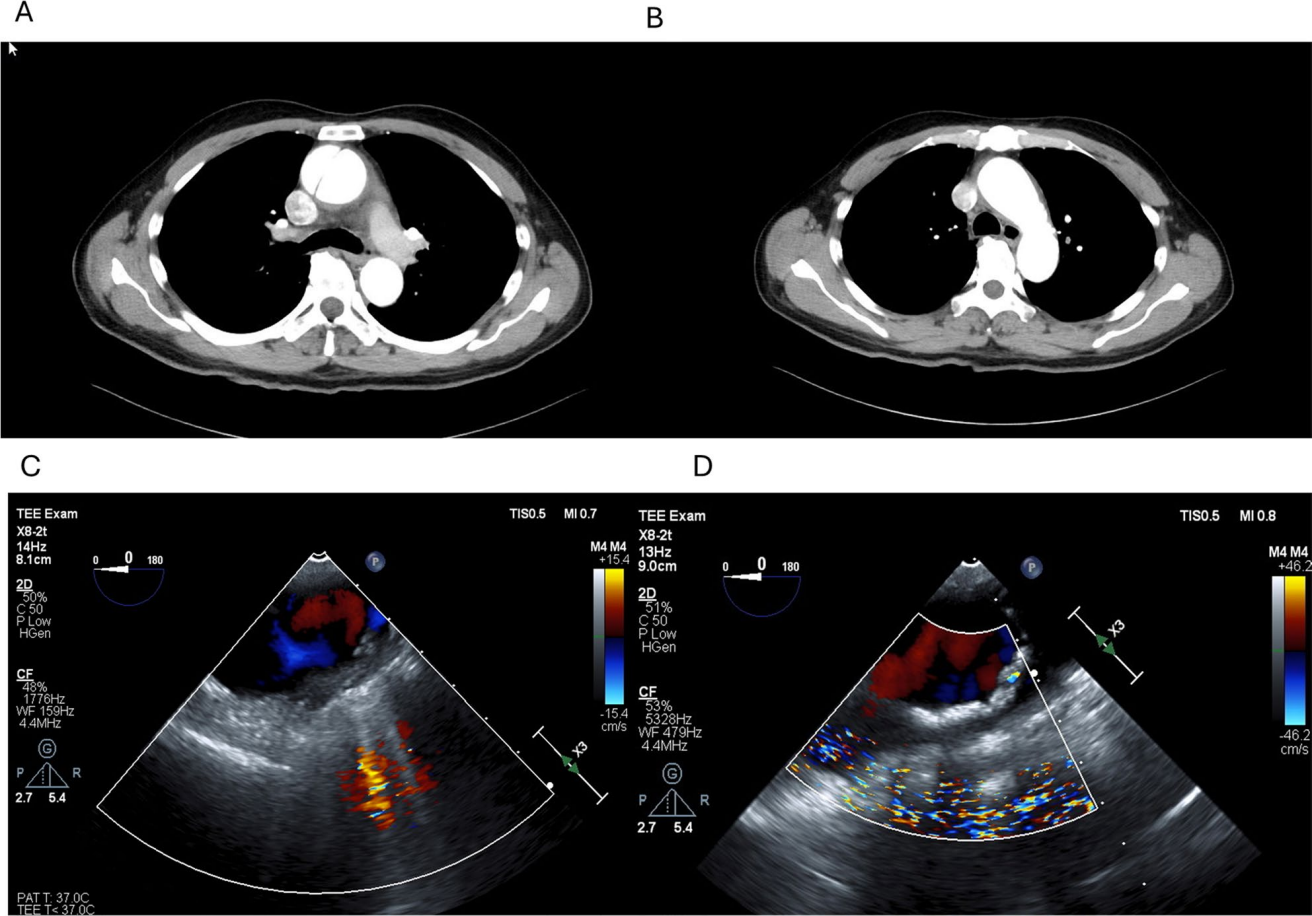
Panels A and B show preoperative contrast-enhanced chest computed tomography images. A patent false lumen is visible in panel A, whereas no dissection is observed in panel B, indicating DeBakey type I dissection. Panel C shows a transesophageal echocardiography (TEE) image of the aortic arch obtained immediately after anesthesia induction, showing no evidence of a false lumen. Panel D shows a TEE image captured at the time of rSO₂ decline, demonstrating the extension of the dissection
